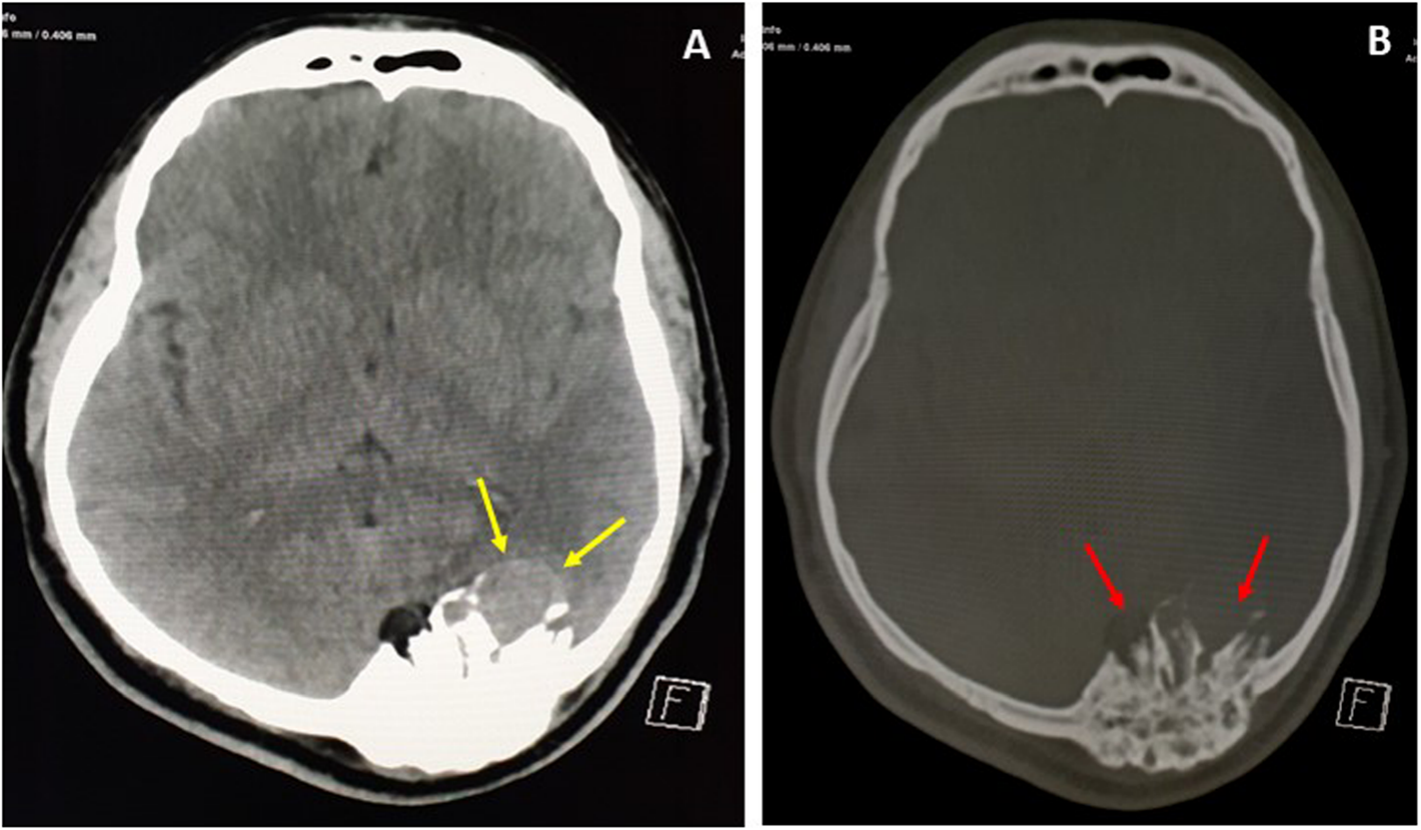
Non-enhanced computed tomography (NECT) scan of brain (a); and bone window (b) showing large left occipital, expansile, lytic lesion with sunburst (Red arrows) appearance, and intracranial extension with soft tissue (Yellow arrows) component
